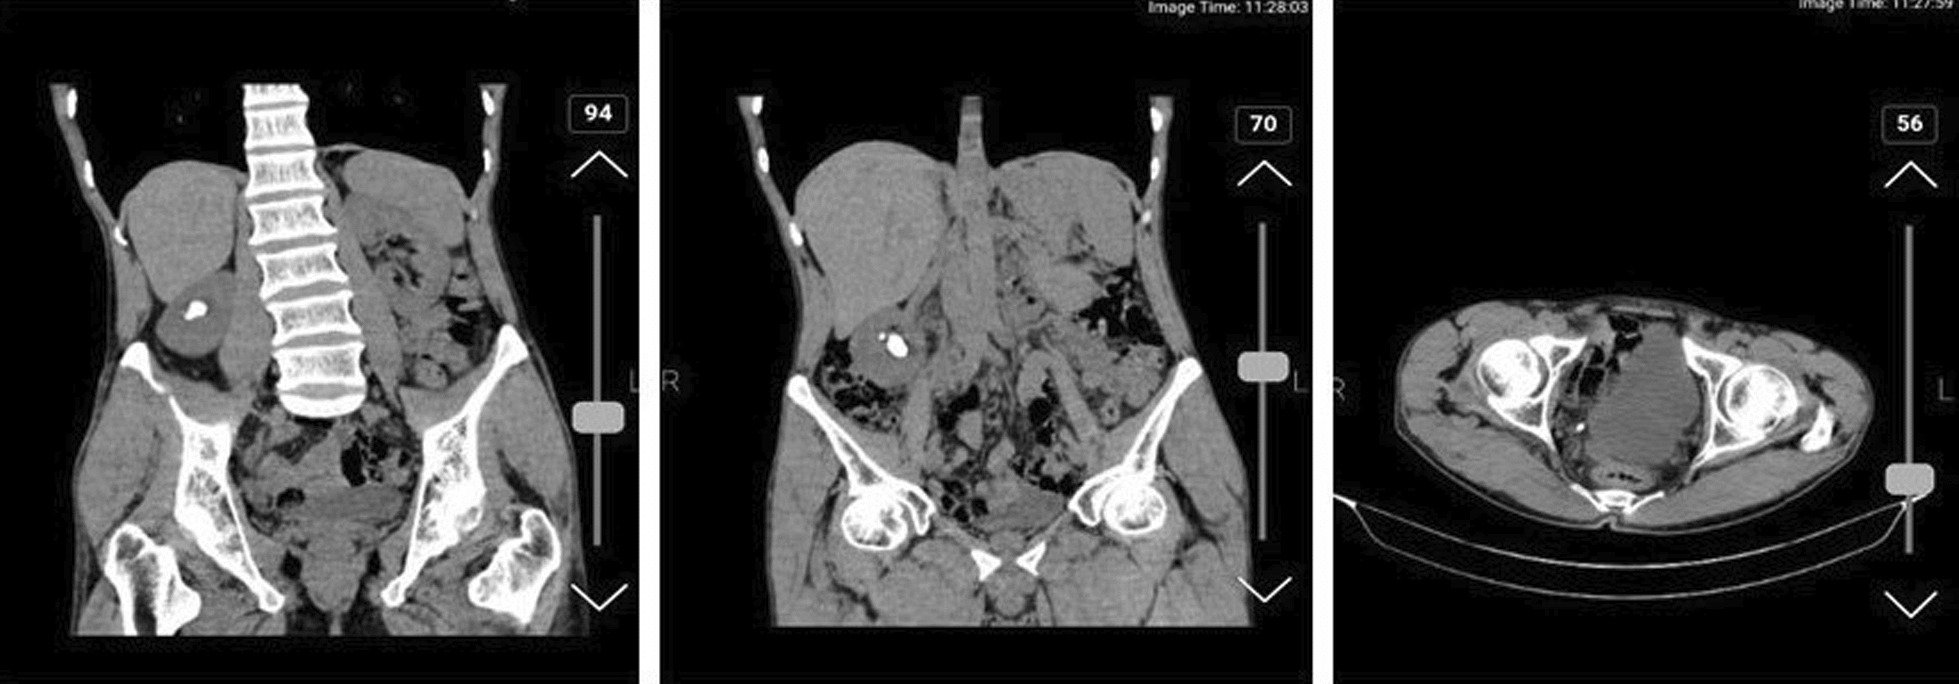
Computed tomography scan of the second case indicating urinary stones in the right kidney and ureter
